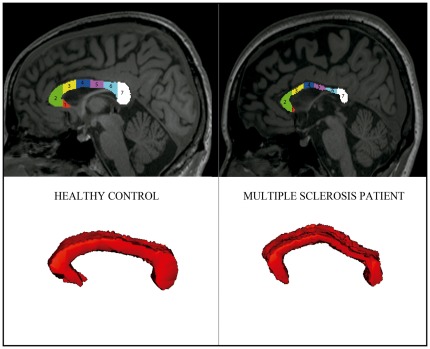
Structural magnetic resonance imaging of the corpus callosum. Area of corpus callosum in midsagittal slice with subdivision in 7 segments, corresponding consecutively to the rostrum, genu, rostral body, anterior midbody, posterior midbody, isthmus, and the splenium (top images) and volume obtained from 11 consecutive central sagittal slices (bottom images). Left images correspond to a healthy control and right images to a multiple sclerosis patient.
